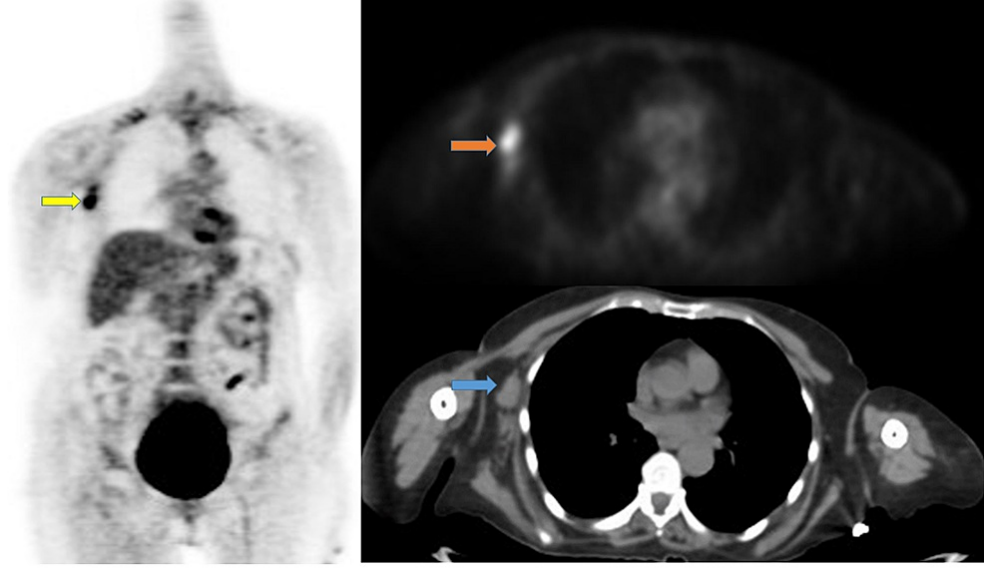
CT PET scan CT: Computer tomography; PET: Positron emission tomography Left-hand side figure panel: the yellow arrow shows hyper-metabolic right axillary adenopathy with a maximum standardized uptake value (SUVmax) of 7.9 Right-hand side figure panel: orange and blue arrows show hyper-metabolic right axillary adenopathy
